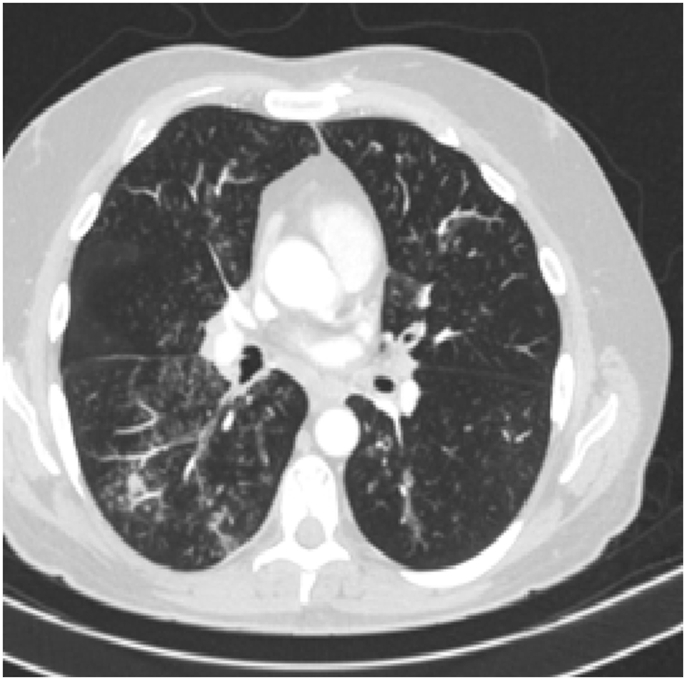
CT chest - lung window, February 2018: bilateral ground-glass opacities with bilateral bronchial wall thickening of both upper lobes and basal segments of the right lower lobe.
